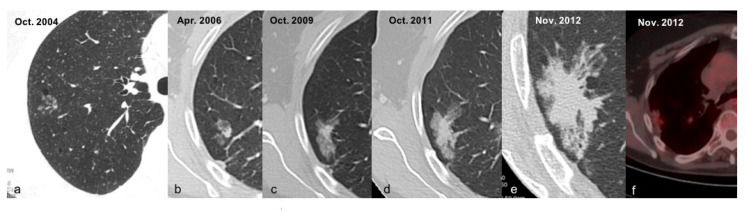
(a–f) This is an example of a PS pre-malignant nodule (a) with evolution in malignant lesion (a–e), confirmed by PET-CT (f), in accordance with changes in the solid component of the nodule.
